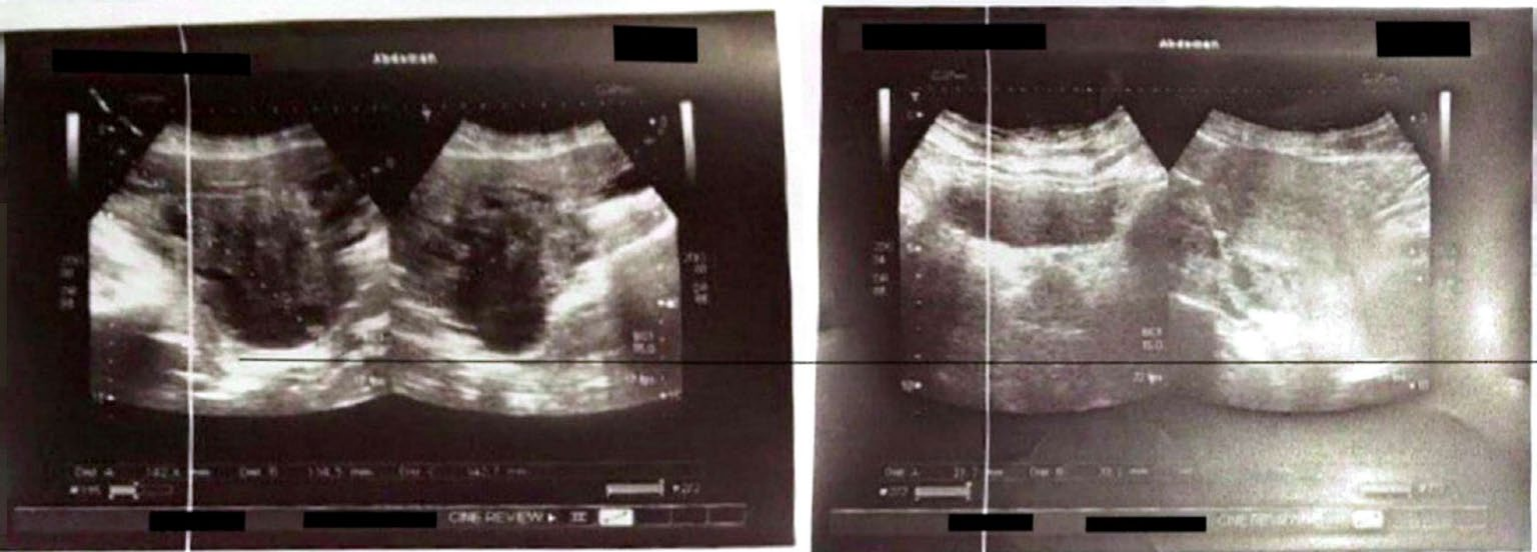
A Pelvic ultrasound shows cervix leiomyoma. The size of the mass is approximately 147 × 145 × 114.5 mm.
